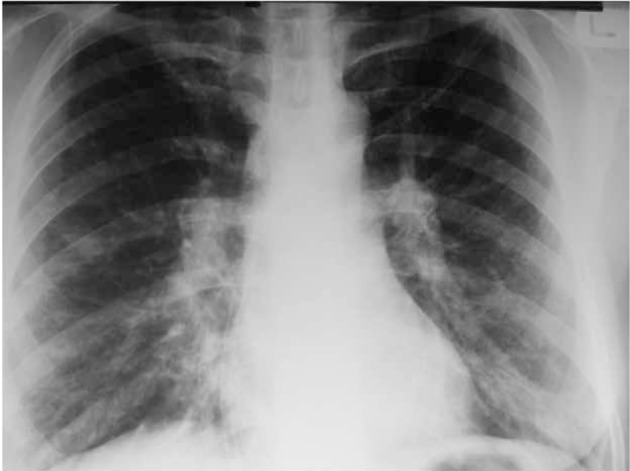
A 23-year-old female with respiratory symptoms and proved H1N1 infection, with crack addiction. Lymphadenopathy is shown on the chest x-ray.
